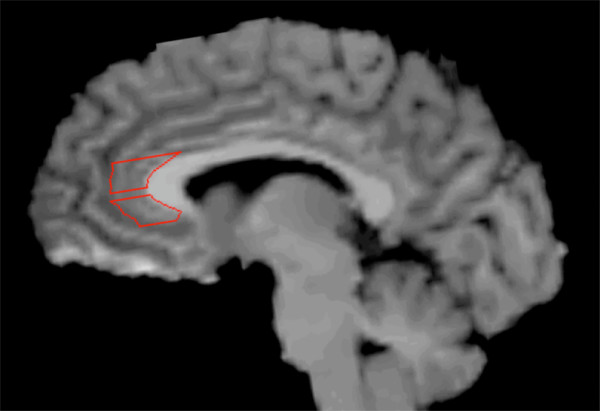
Sagittal image of single-subject magnetic resonance image with pgPFC (top) and sgPFC (bottom) ROIs outlined. Despite its appearance, pgPFC and sgPFC ROIs are drawn on consecutive transaxial slices. pgPFC = pregenual prefrontal cortex; ROIs = regions of interest; sgPFC = subgenual prefrontal cortex.
